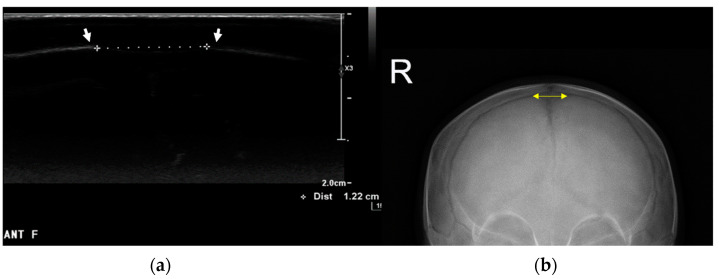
Measurement of anterior fontanelle size using ultrasonography (a) and an anterior–posterior (AP) radiograph of the skull (b). (a) The size of the anterior fontanelle was defined as the longest transverse distance between the skull bones, as indicated by the white arrows in the ultrasound image; (b) similarly, in the skull AP radiograph, the size was determined by the longest transverse distance between the ends of each coronal suture, marked by the yellow arrows.
